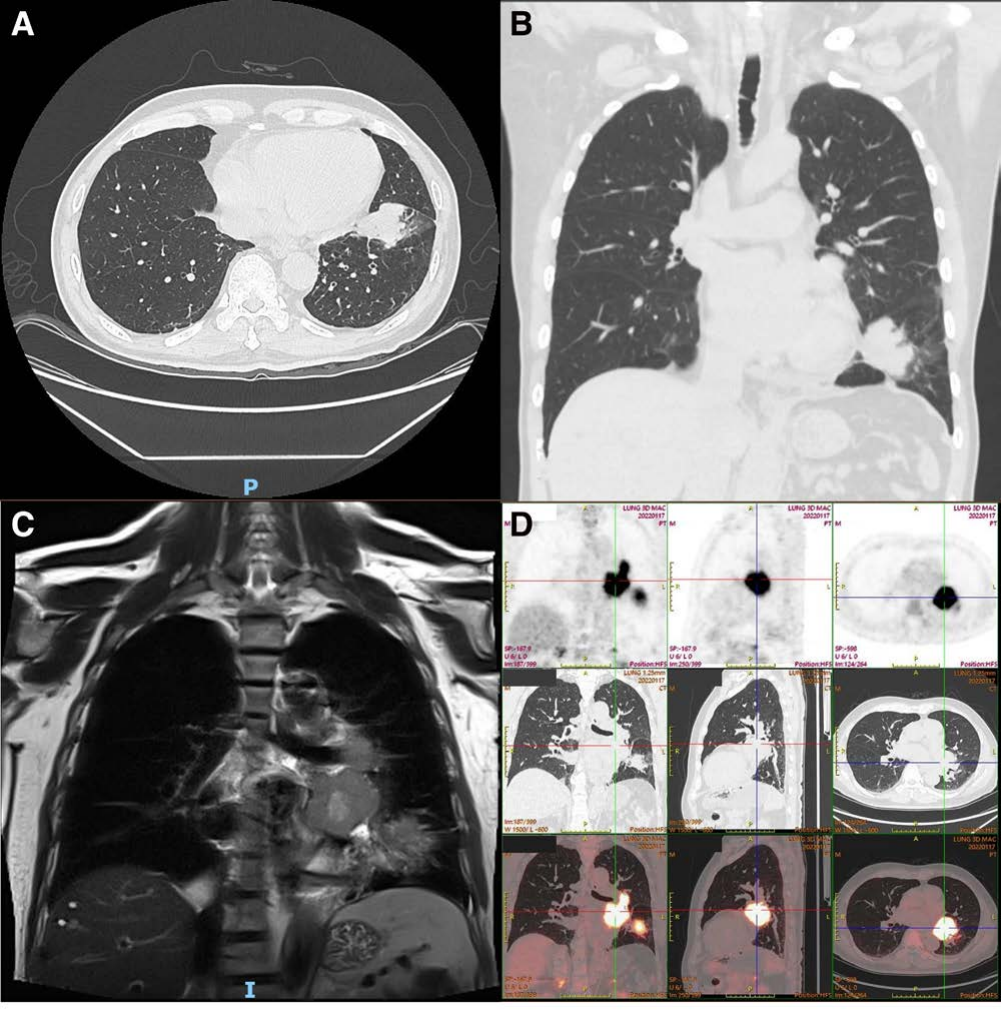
Imaging findings in thorax. (A) CT from transverse view. (B) CT from coronal view. (C) MRI from coronal view. (D) PET-CT. CT = computed tomography, MRI = magnetic resonance imaging, PET-CT = positron emission tomography-computed tomography.
